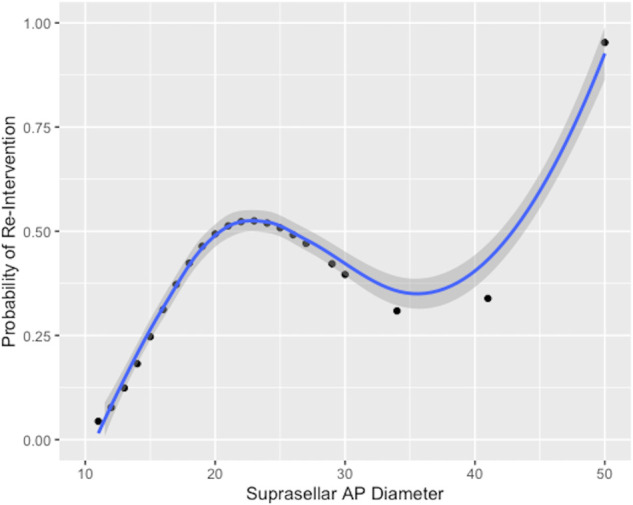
Graphical representation of the basic spline logistic regression model, demonstrating the variation in the probability of reintervention after intraoperative MRI with increasing suprasellar AP diameter. Gray shading indicates 95% CI of the estimate. AP, anterior-posterior.
